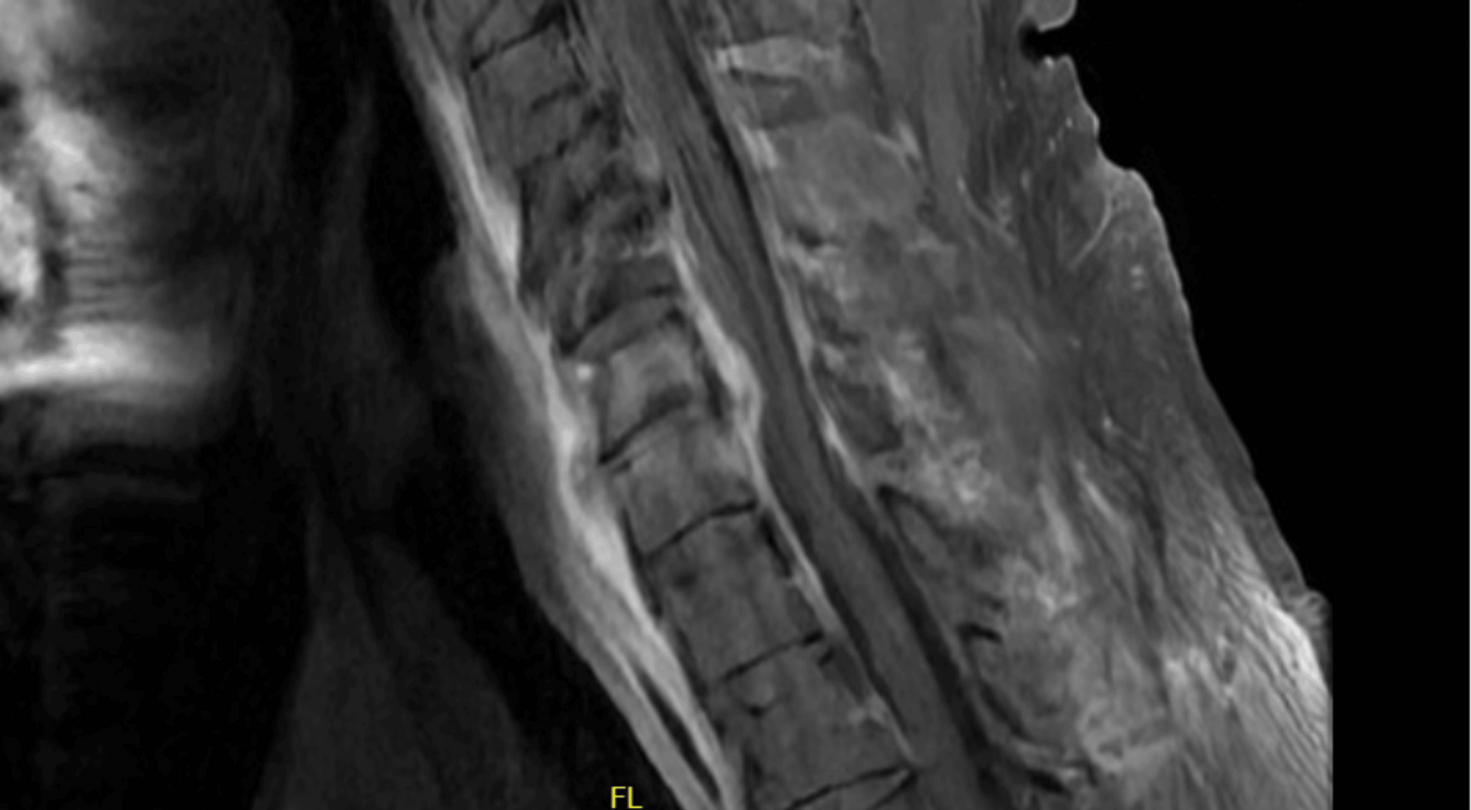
Cervical spinal CT revealing a developing epidural abscess with osteomyelitis at C6/7.
